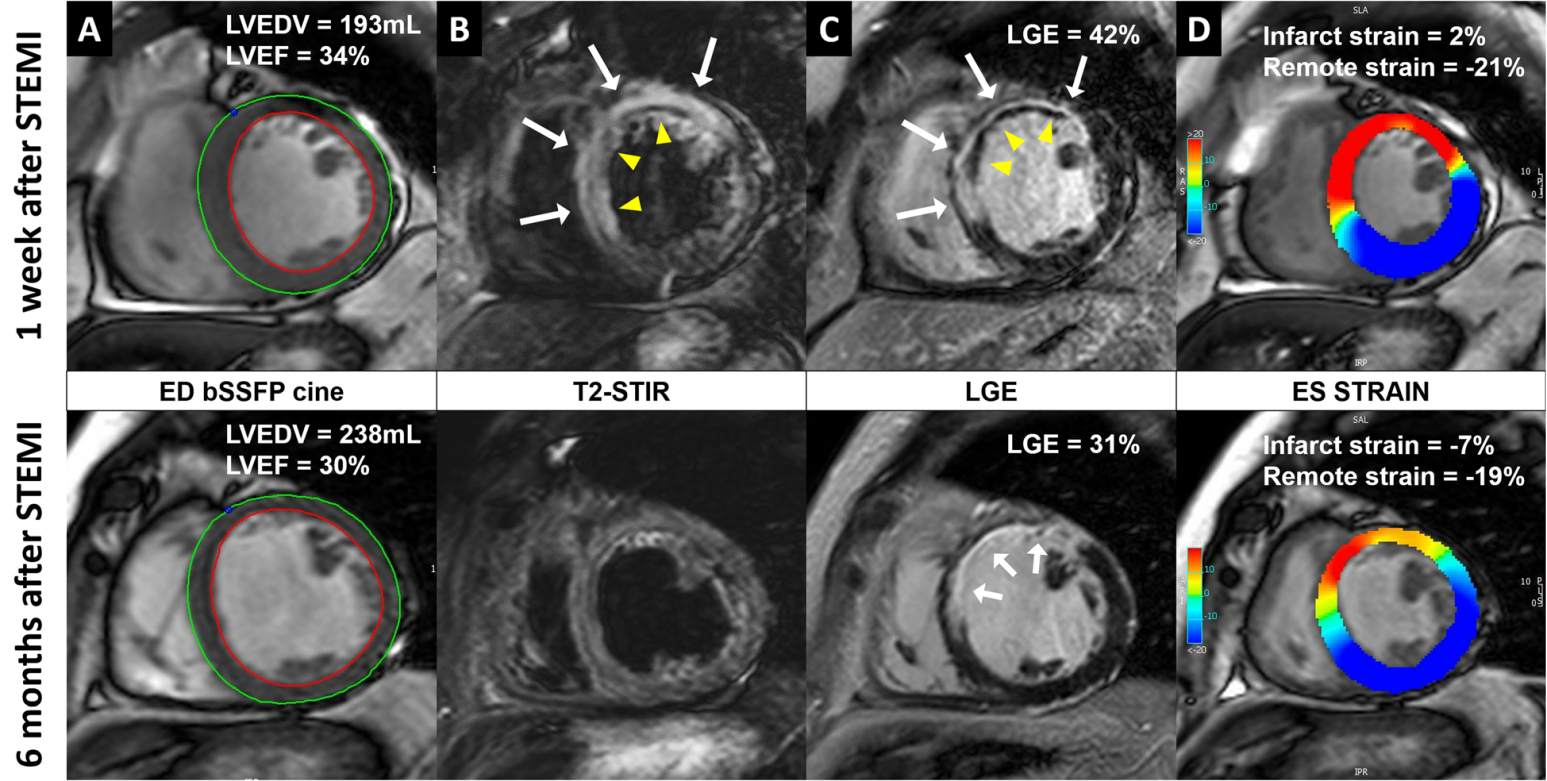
A patient with a proximal LAD infarct receiving standard STEMI treatment. a, balanced steady state free precession (bSSFP) end-diastolic images with endo- and epi-cardial contours. The patient developed adverse left ventricular (LV) remodeling (defined as ≥ 20% increase in LV end-diastolic volume (LVEDV) and had a slight reduction in LV ejection fraction (LVEF) at 6 months after STEMI. b, T2-weighted short tau inversion recovery (STIR) images, showing the presence of edema (white arrows) and intramyocardial hemorrhage (IMH) (yellow arrowheads) at 1-week after STEMI. c, late gadolinium enhancement (LGE) images, showing the presence of acute ischemic injury (white arrows) with microvascular obstruction (MVO, yellow arrowheads) at 1 week after STEMI and infarct scar (white arrows) at 6-months. d, end-systolic bSSFP images with feature-tracking circumferential LV strain overlay. At 6 months the infarct zone circumferential strain improved despite the presence of a huge infarct with IMH and MVO in the acute phase, while the remote zone strain slightly declined bSSFP, balanced steady state free precession; ED, end-diastolic; ES, end-systolic; IMH, intramyocardial hemorrhage; LAD, left anterior descending coronary artery, LGE, late gadolinium enhancement; LV, left ventricular; LVEDV, left ventricular end-diastolic volume; LVEF, left ventricular ejection fraction; MVO, microvascular obstruction; SSFP, steady-state free precession; STEMI, ST-segment elevation myocardial infarction; STIR, short tau inversion recovery.
